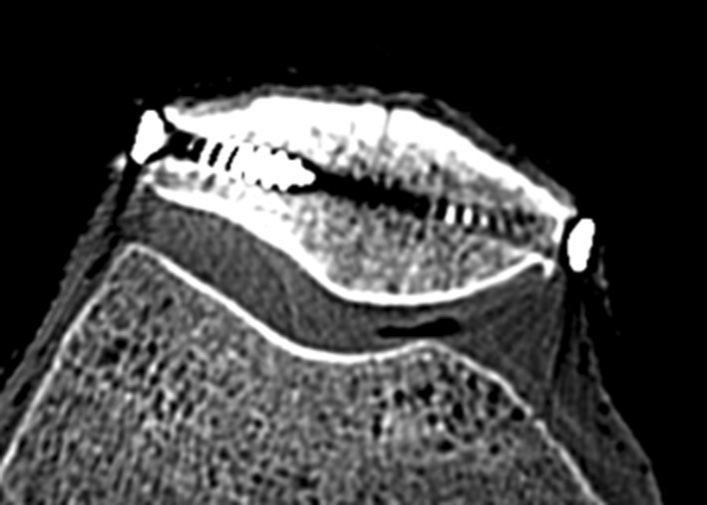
Positioning of patellar screws. We ensured that screws were positioned in one line, with their tips pointing together and to the center of the patella. The CT scan shows a transverse section of a typical patella at the screw level.
